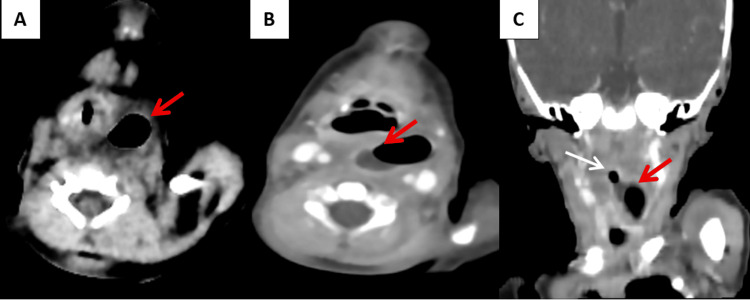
Cervical CT scan images Cervical CT scan without (A) and with contrast administration (B–C), in axial sections (A–B) and coronal reconstruction (C) reveals a well-defined left basi-cervical collection with an air-fluid level (red arrow). The collection exhibits a thickened wall with post-contrast enhancement and is in intimate contact with the esophagus (white arrow).
